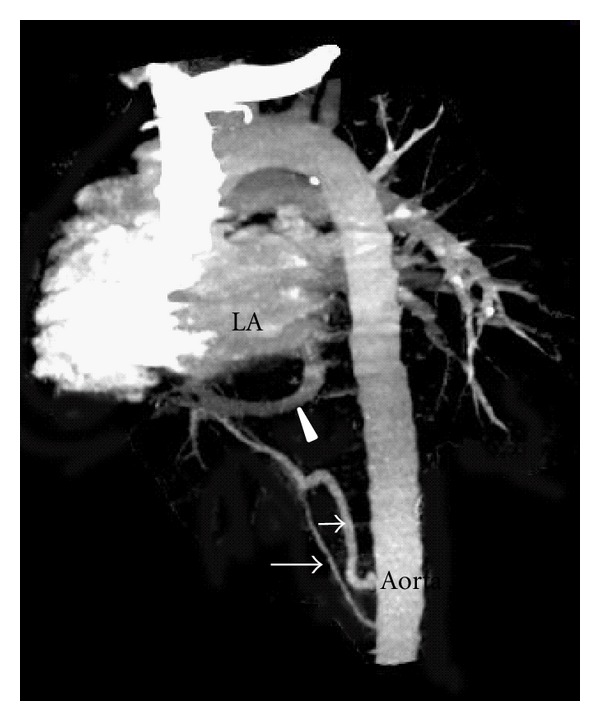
CT angiography volume rendered image depicts two anomalous arterial supply to the sequestration (arrows) and meandering right inferior pulmonary vein (arrow head).
